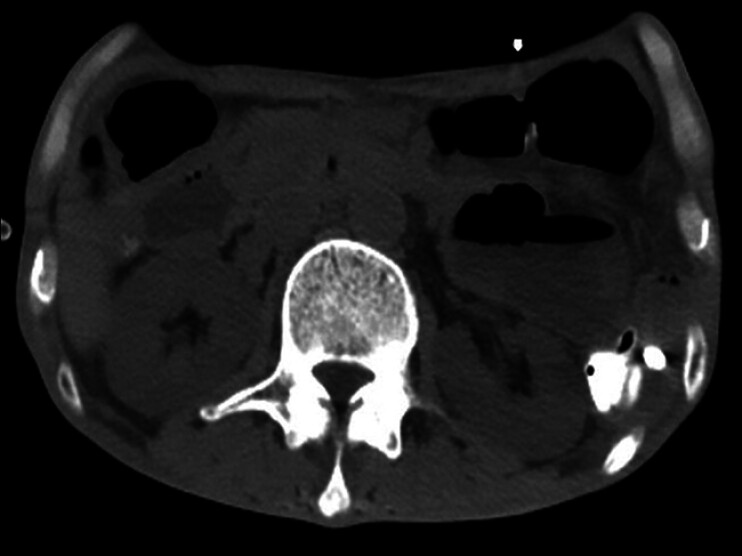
Computed tomography indicated a significant reduction in duodenal dilation and fluid accumulation.
